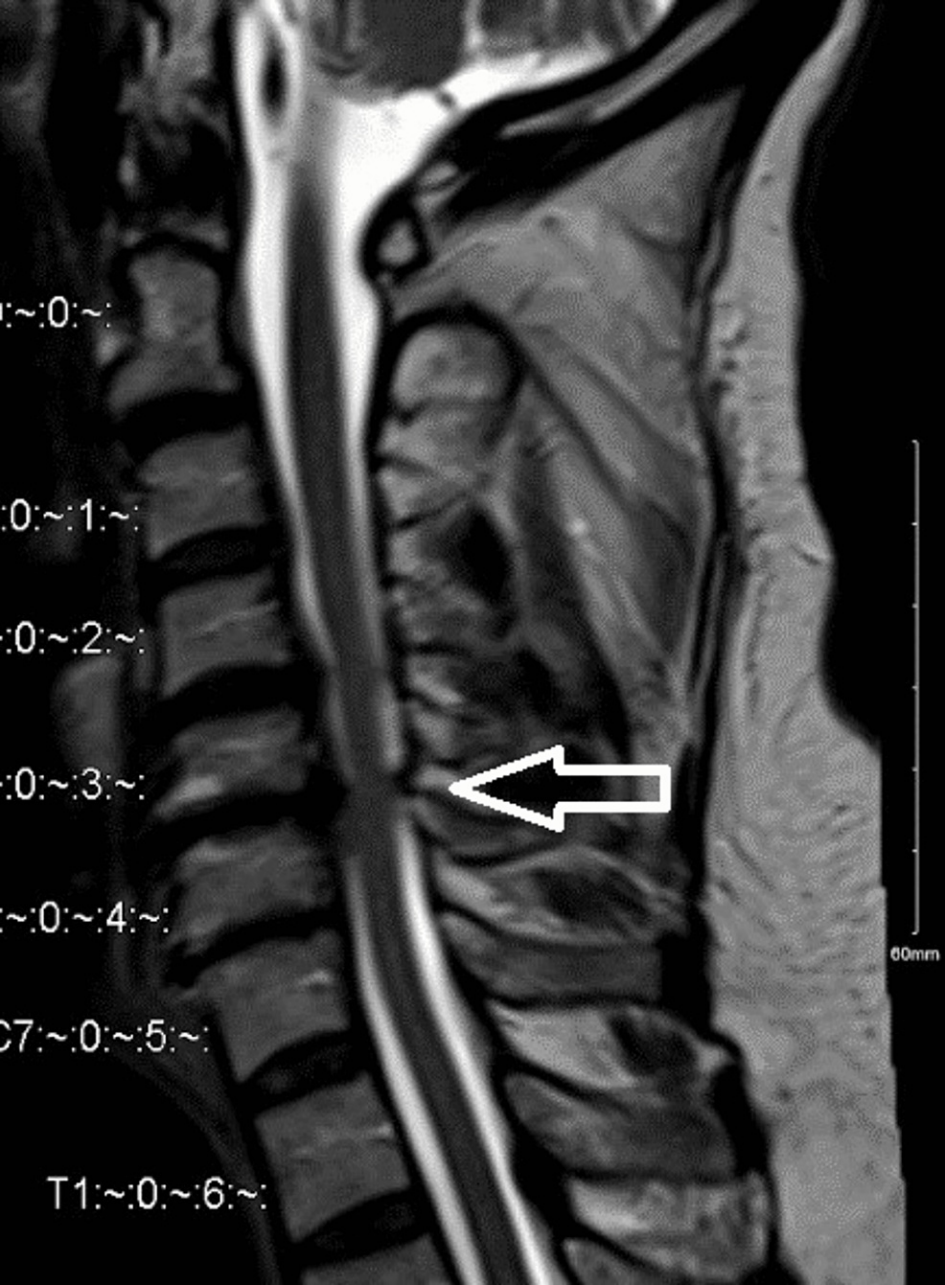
Magnetic resonance imaging scan sagittal view. Revealed multiple level of cervical spine degenerative changes more at C5/6 causing sever canal stenosis and myelomalacia.
